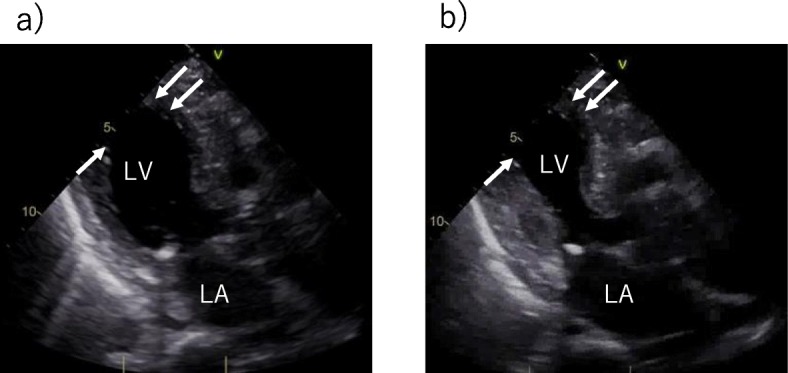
Echocardiography at presentation (a: diastole b: systole). The apical region of the left ventricle was akinetic (white arrows), and there was no pericardial effusion.(LA, Left Artium; LV, Left Ventricle)
